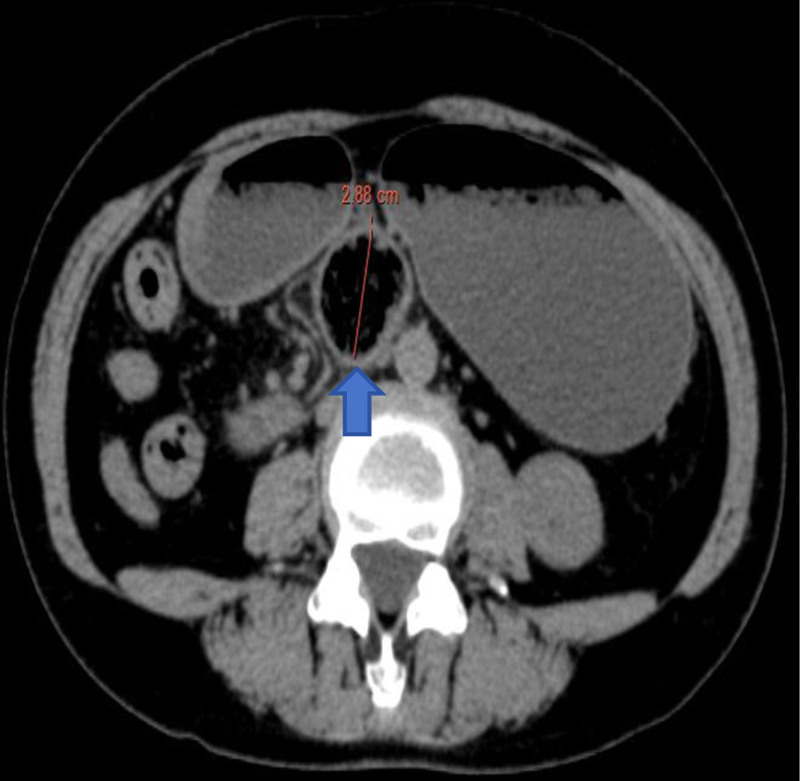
Location of the stone on abdominal CT (axial position).
